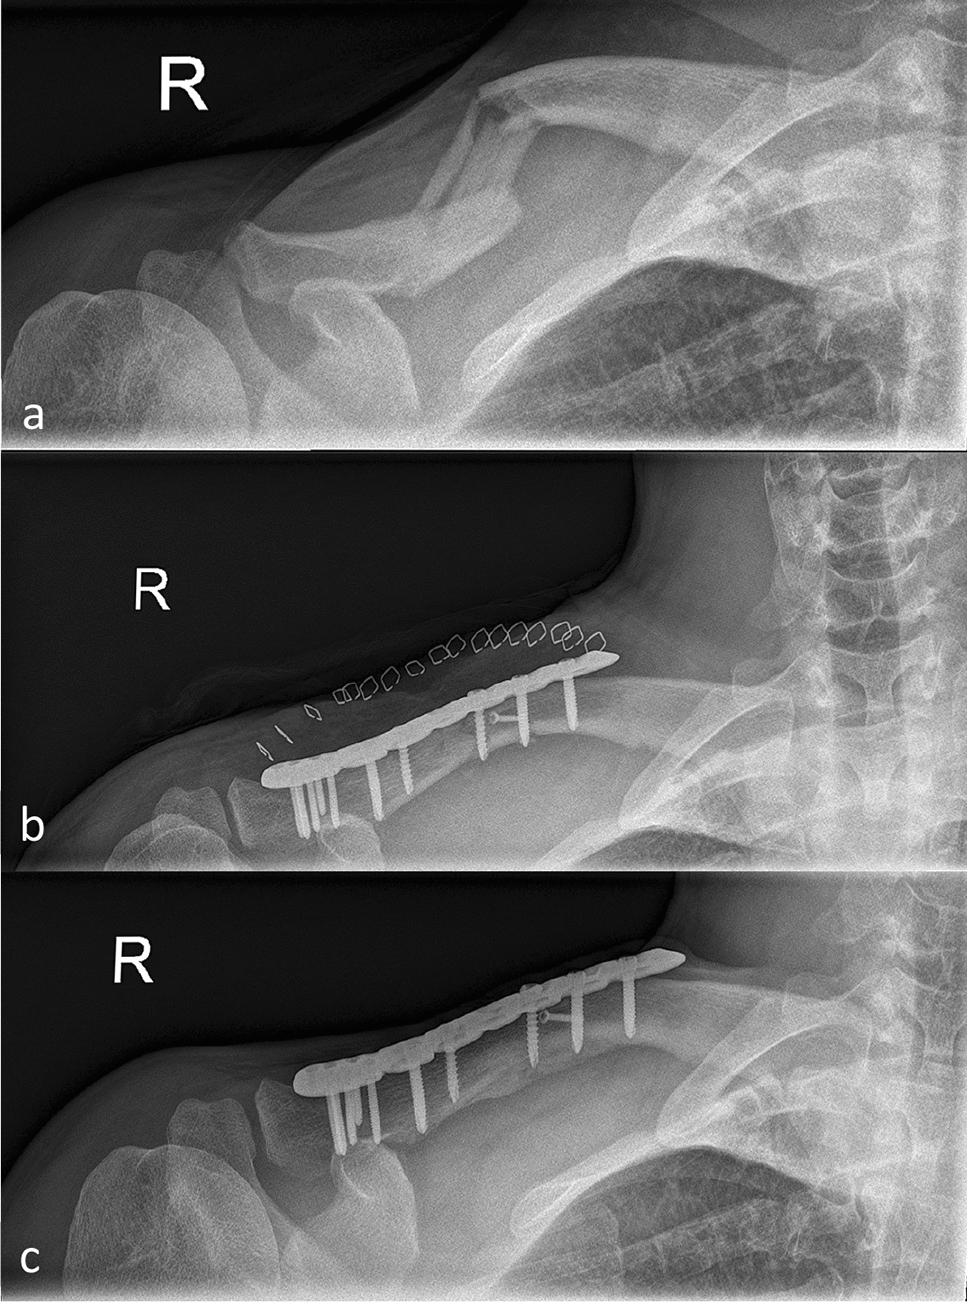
X-ray images of a fragmentary (OTA 2.C) midshaft clavicle fracture treated with 2.0-mm cortical screw augmentation and locking compression plate. Preoperative (a), postoperative (b) and images after fracture consolidation
